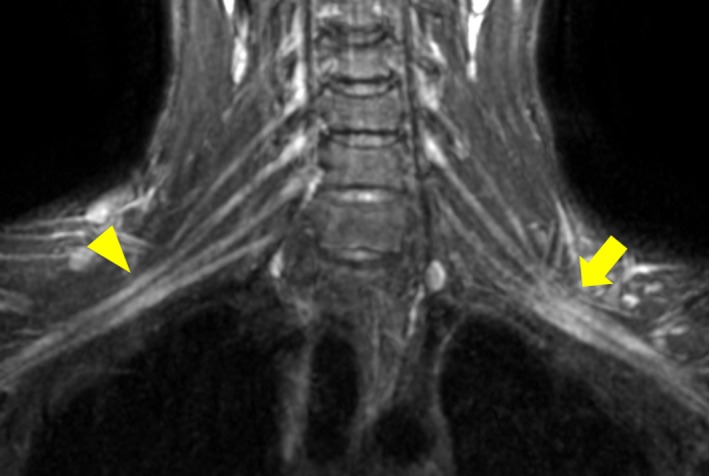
Magnetic resonance imaging of the brachial plexus. Maximum intensity projection imaging of short TI inversion recovery (STIR) (inversion time/repetition time/echo time = 160/2,838/69 ms) indicated enlargement and hyperintensity of bilateral brachial plexuses, predominantly on the left side (arrow: left side, arrowhead: right side)
